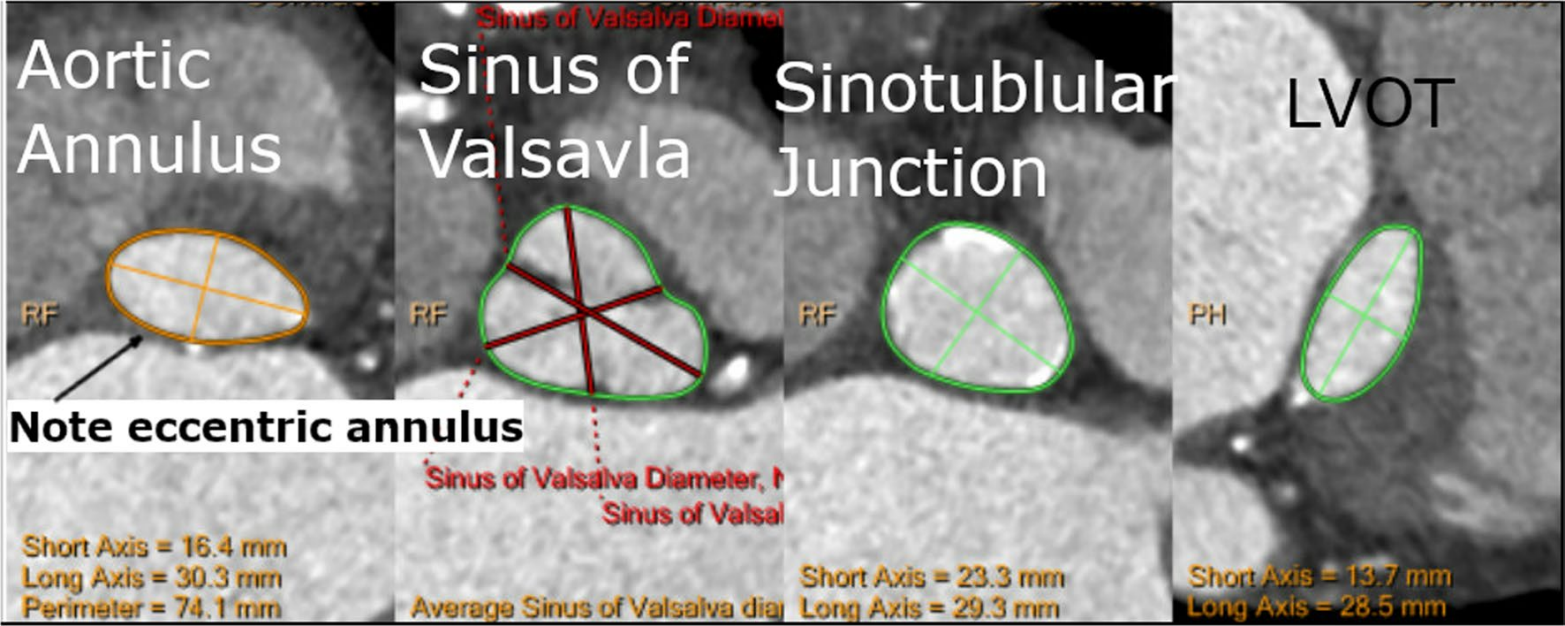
Patient 3 pre-operative CT scan
